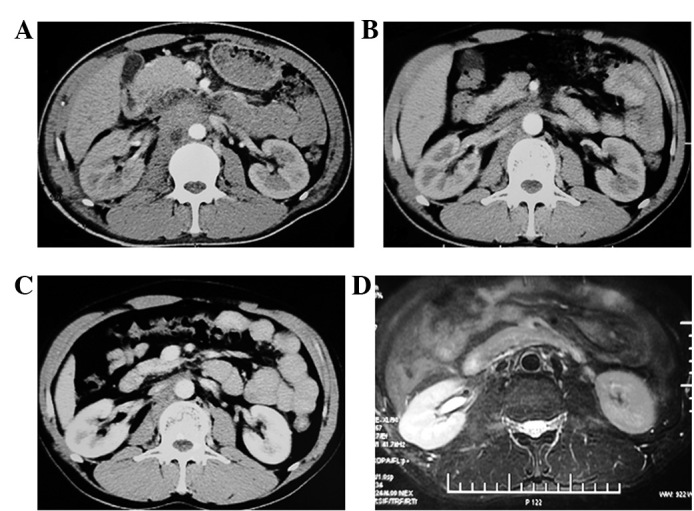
CT scan images and MRI showing the stages of the physiological changes of the abdomen following the treatments of prednisone. (A) The first month of the treatment. The CT scan showed that the mass was the same size, but the clinical symptoms, including the back pain, were reduced. (B) The third month of the treatment of prednisone. The CT scan showed that the mass was much smaller than in the first month. (C) The sixth month of the treatment of prednisone. The CT scan showed that the border of surrounding tissues was clear and that the mass was further reduced in size. The clinical symptoms disappeared. (D) The last month of the treatment of prednisone. MRI showed that the mass had almost disappeared and that the outlines of the abdominal aorta and inferior vena cava were clear.
